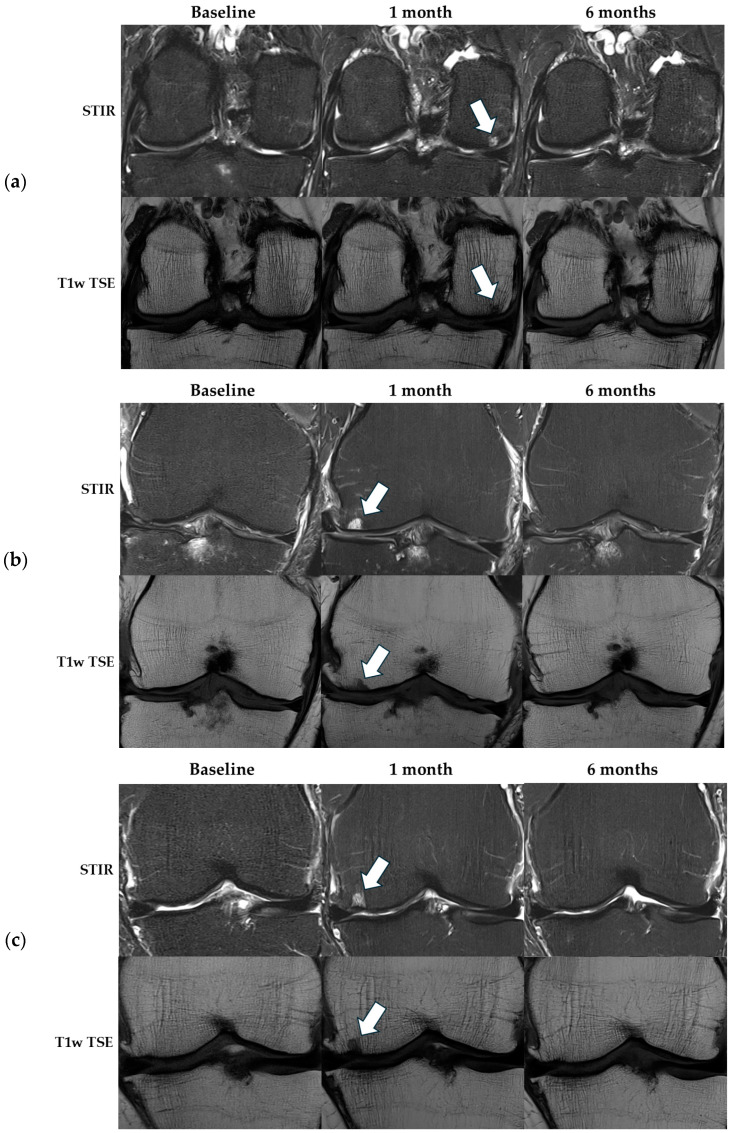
Ischemic-like lesions on MRI. Three cases of nonspecific ischemic-like lesions one month after GAE (white arrows), all completely resolved by six months. The three participants were embolized in the following areas: (a) medial side of the right knee, (b) medial and lateral side of the right knee, and (c) medial side of the left knee. In all three cases, the lesions corresponded to the treated areas.
